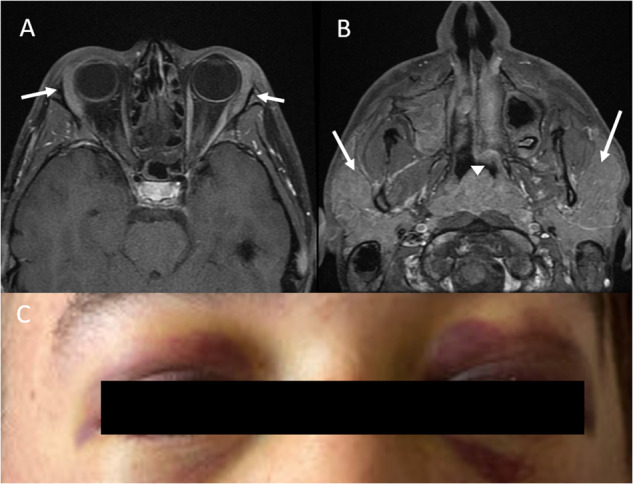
Imaging Findings in CNS BPDCN. Axial MRI of the face of a teenage male with BPDCN shows diffusely enlarged lacrimal glands (arrows in A), diffusely enlarged parotid glands (arrows in B), and a diffusely enlarged adenoid (arrowhead in B). CT of the neck, chest, abdomen, and pelvis reveals multicompartmental lymphadenopathy (involving the cervical, axillary, hilar, mediastinal, periportal, retroperitoneal, and iliac chain and inguinal lymph nodes) and hepatosplenomegaly (not shown). Brain and spine MRI reveal no leptomeningeal disease. Periorbital ecchymosis (“raccoon eyes”) at presentation in a patient later diagnosed with BPDCN (C).
